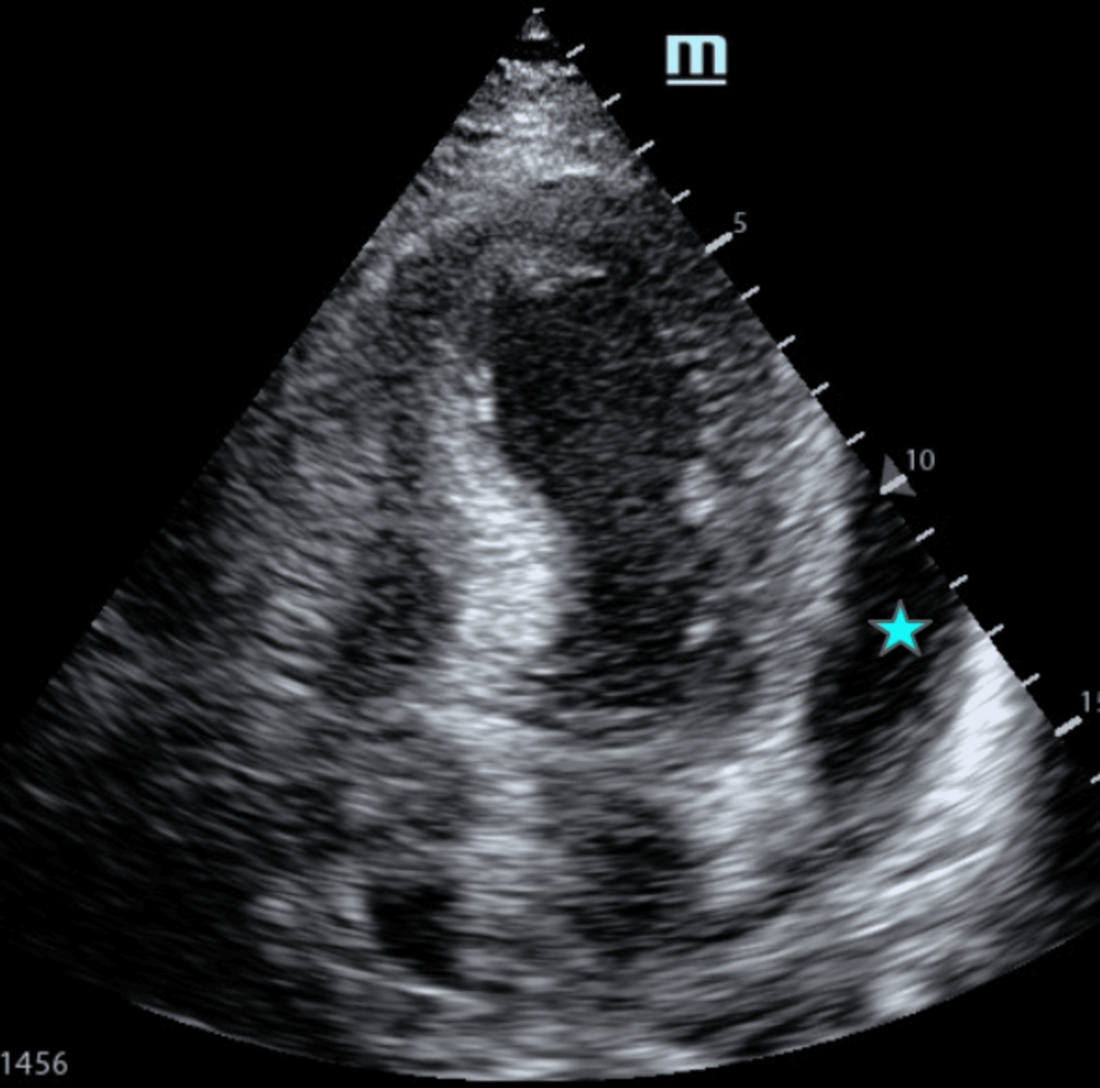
Apical four-chamber view demonstrating a left ventricular aneurysm and an intracavitary thrombus Transthoracic echocardiography in the apical four-chamber view demonstrating apical aneurysmal dilation of the left ventricle with an associated intracavitary thrombus. A circumferential pericardial effusion is also noted (blue asterisk).
